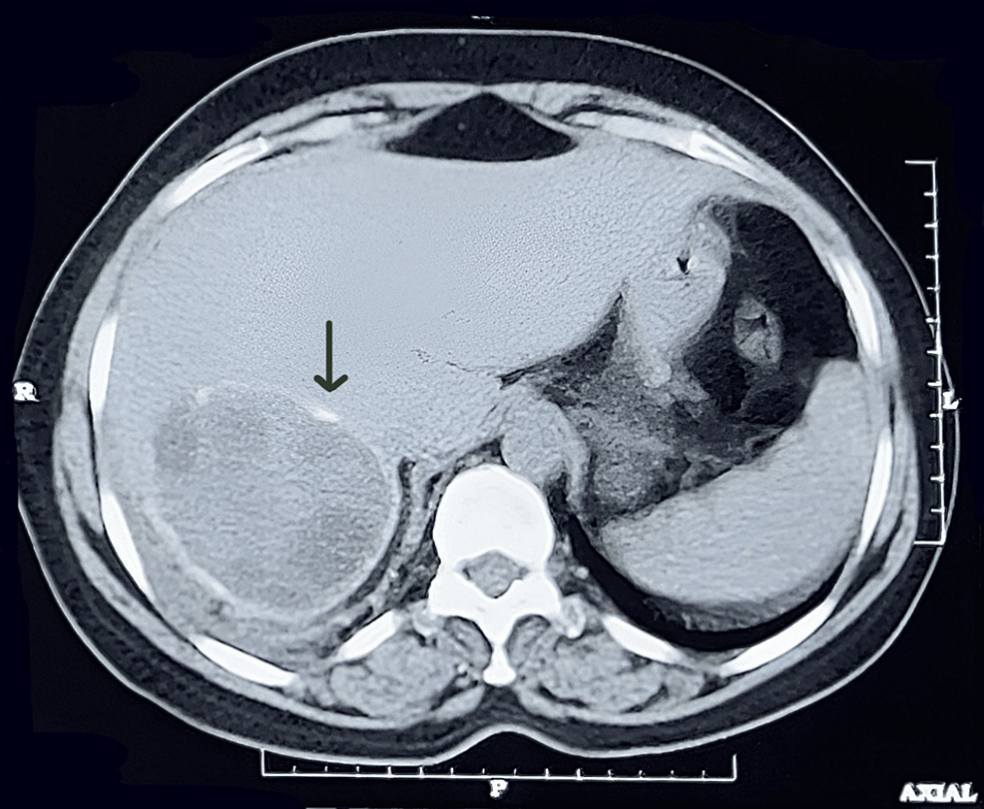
Contrast-enhanced computed tomography scan showing a well-defined lesion involving segments VI and VII of the liver containing a few daughter cysts
